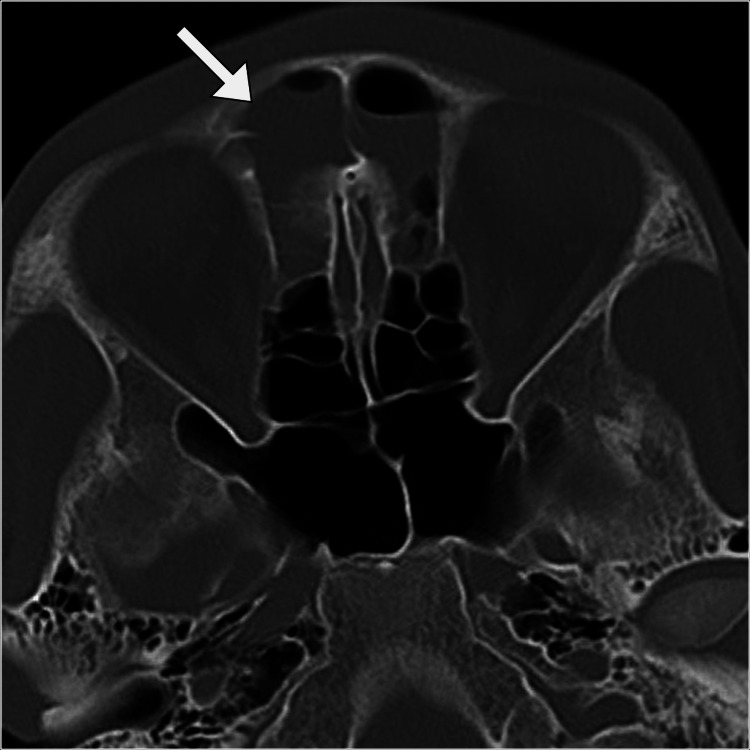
Axial CT head image displaying mucosal thickening with an air-fluid level in the frontal sinuses (arrow), indicative of acute sinusitis. CT: computed tomography.
